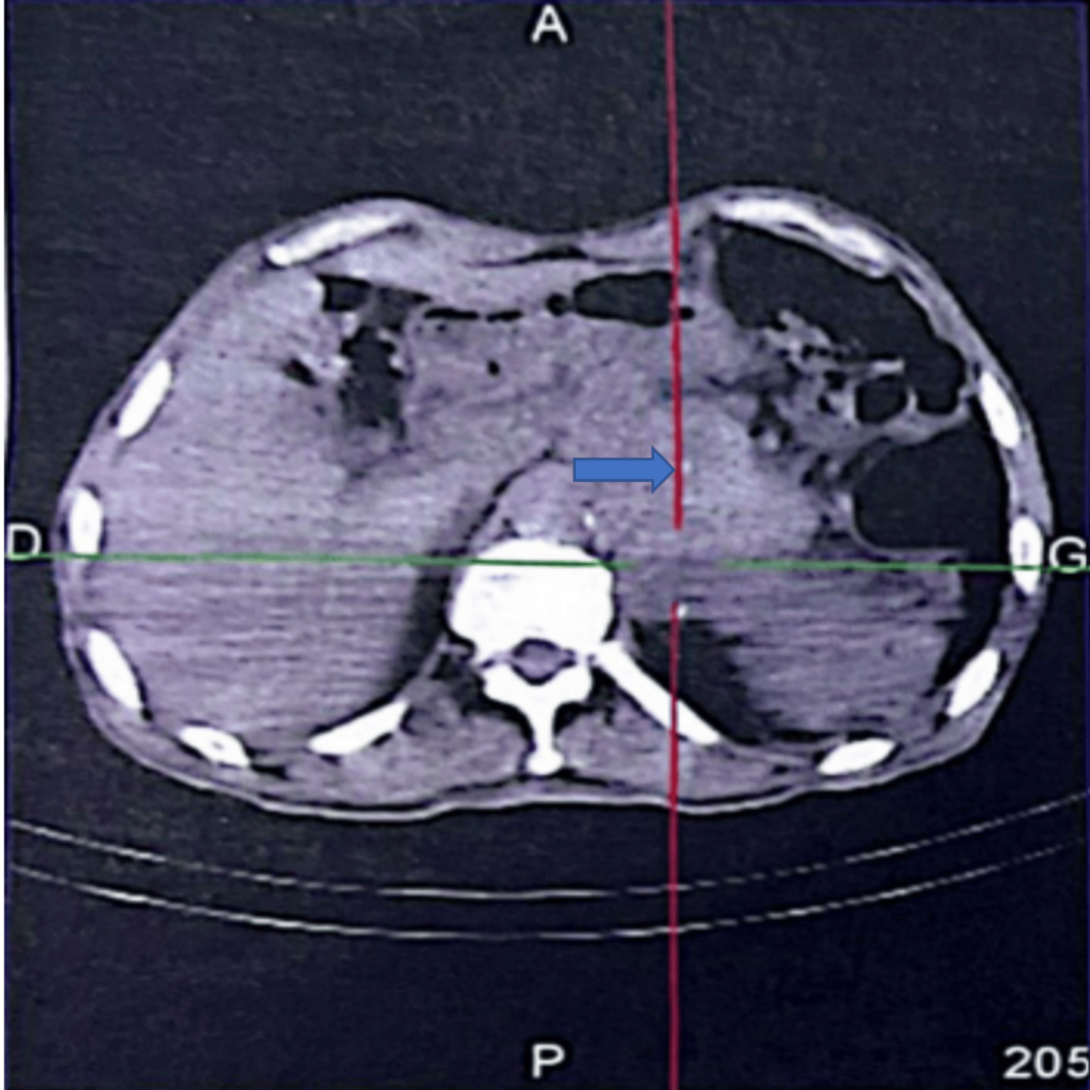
¹²³I-MIBG scintigraphy showing focal radiotracer uptake in the left adrenal region, consistent with pheochromocytoma ¹²³I-MIBG: iodine-123 metaiodobenzylguanidine
